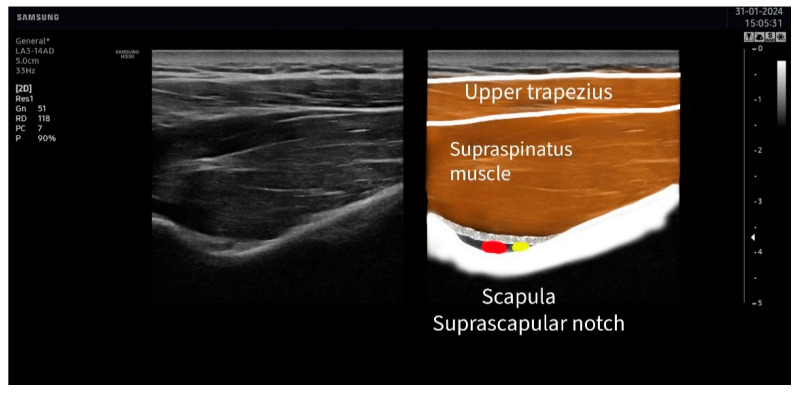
Ultrasound identification of the suprascapular nerve (in yellow) lateral to the artery (in red) at the suprascapular notch. The imaging shows the upper trapezius muscle as the most superficial muscle located over the supraspinatus muscle. The suprascapular notch is located in the deepest part of the suprascapular fossa.
